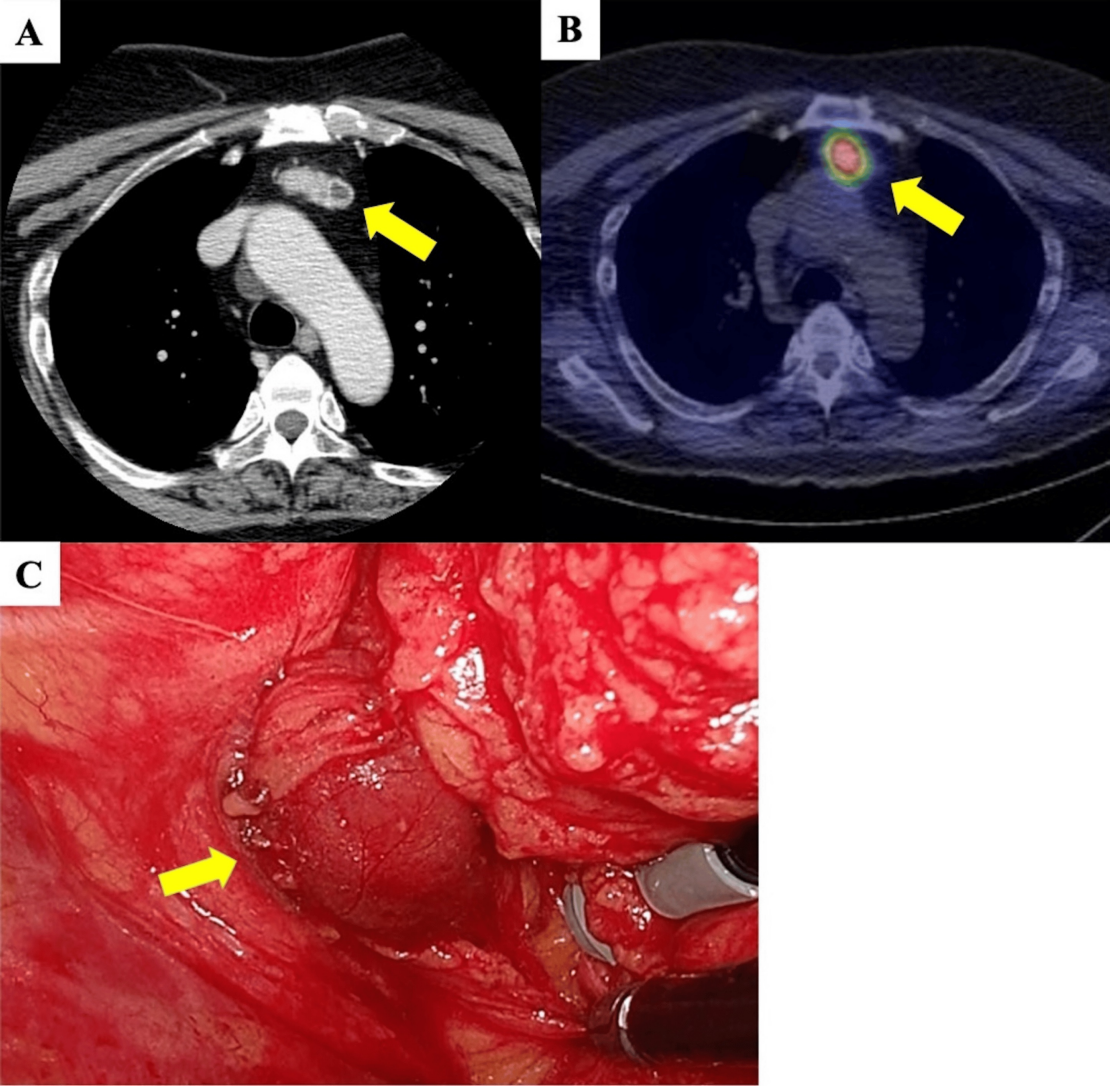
Chest computed tomography (CT) and technetium-99m-methoxy-isobutyl-isonitrile (99mTc-MIBI) scintigraphy and intraoperative findings of the patient (A) Chest CT displayed a 3.3 x 1.4-cm solid mass containing a cyst in the anterior mediastinum (yellow arrow); (B) 99mTc-MIBI scintigraphy displayed increased uptake within the mass (yellow arrow); (C) A round tumor was observed in the anterior mediastinum (yellow arrow).
